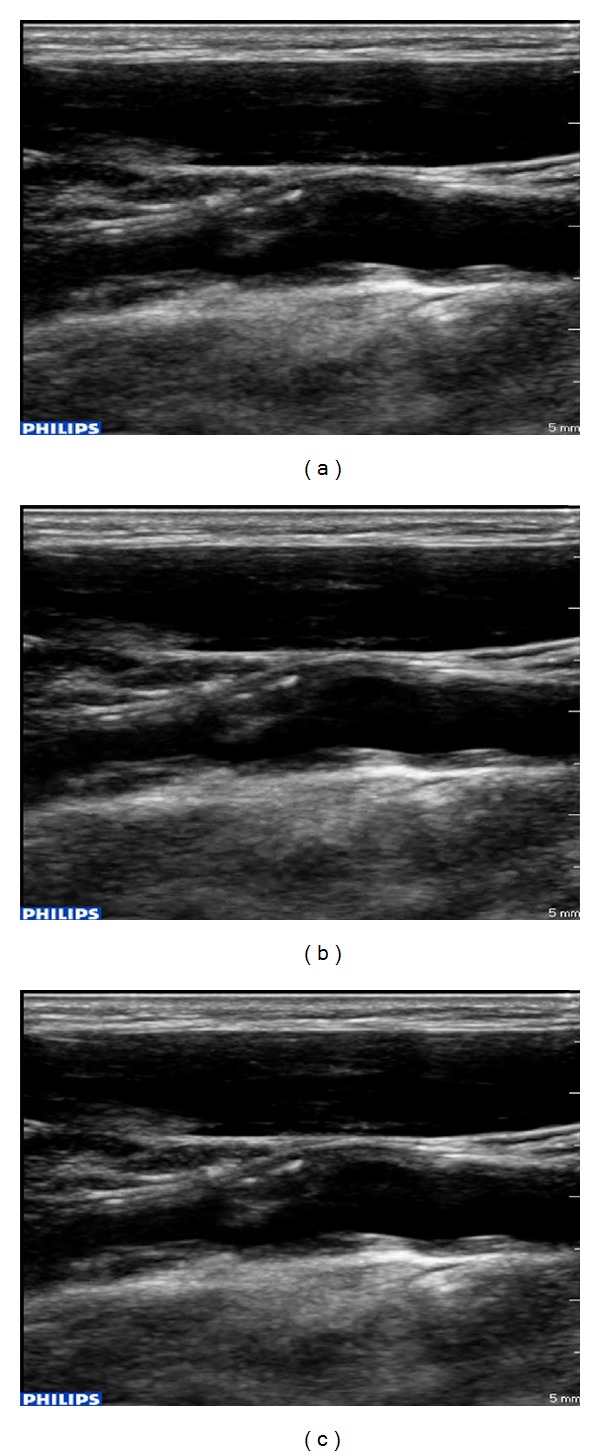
Video image examples of a typical ultrasound video at the acquired, 560 × 416 video resolution. (a) Original ultrasound video, (b) scenario 1 (WLAN): 560 × 416 @ 768 kbps, PSNR: 39.9 dB, and (c) scenario 2 (HSPA): 560 × 416 @ 768 kbps, PSNR-VFD: 39.8 dB.
